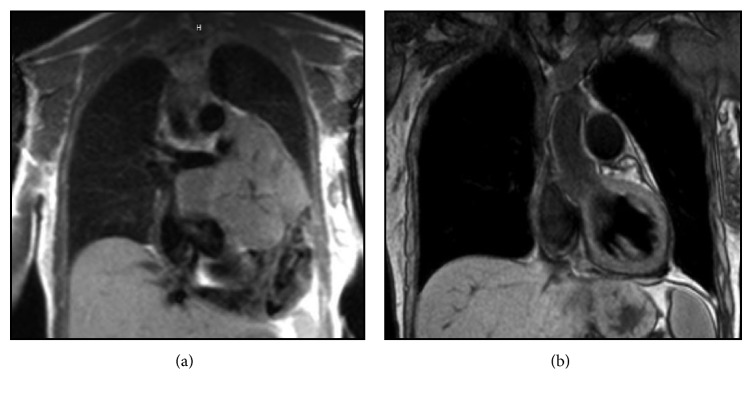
Baseline cardiac MRI shows a large, heterogeneous mass in the pericardial space with a large effusion and compression of the left atrium (a). Neoadjuvant chemotherapy with doxorubicin/ifosfamide ×12 cycles and gemcitabine/docetaxel ×6 cycles resulted in near complete response (b).
